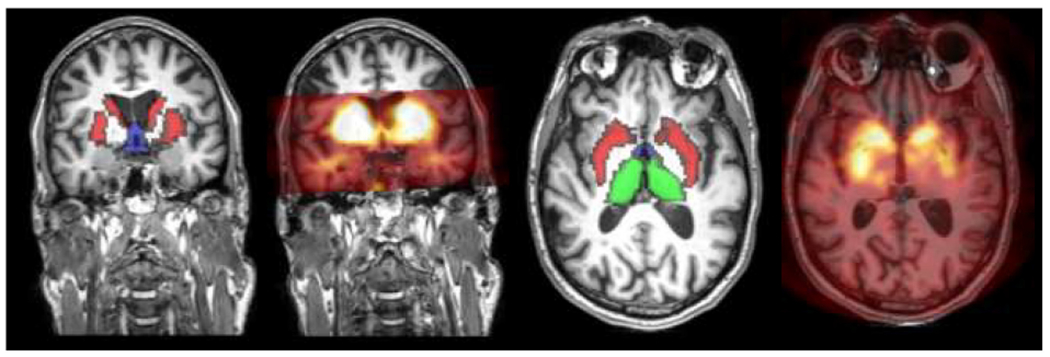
Representative T1w anatomical brain MRI scans overlaid with co-registered SPECT images and ROI masks. Striatal (red mask), hypothalamic (blue mask), and thalamic (green mask) uptake of the radiotracer 123I-FP-CIT.
